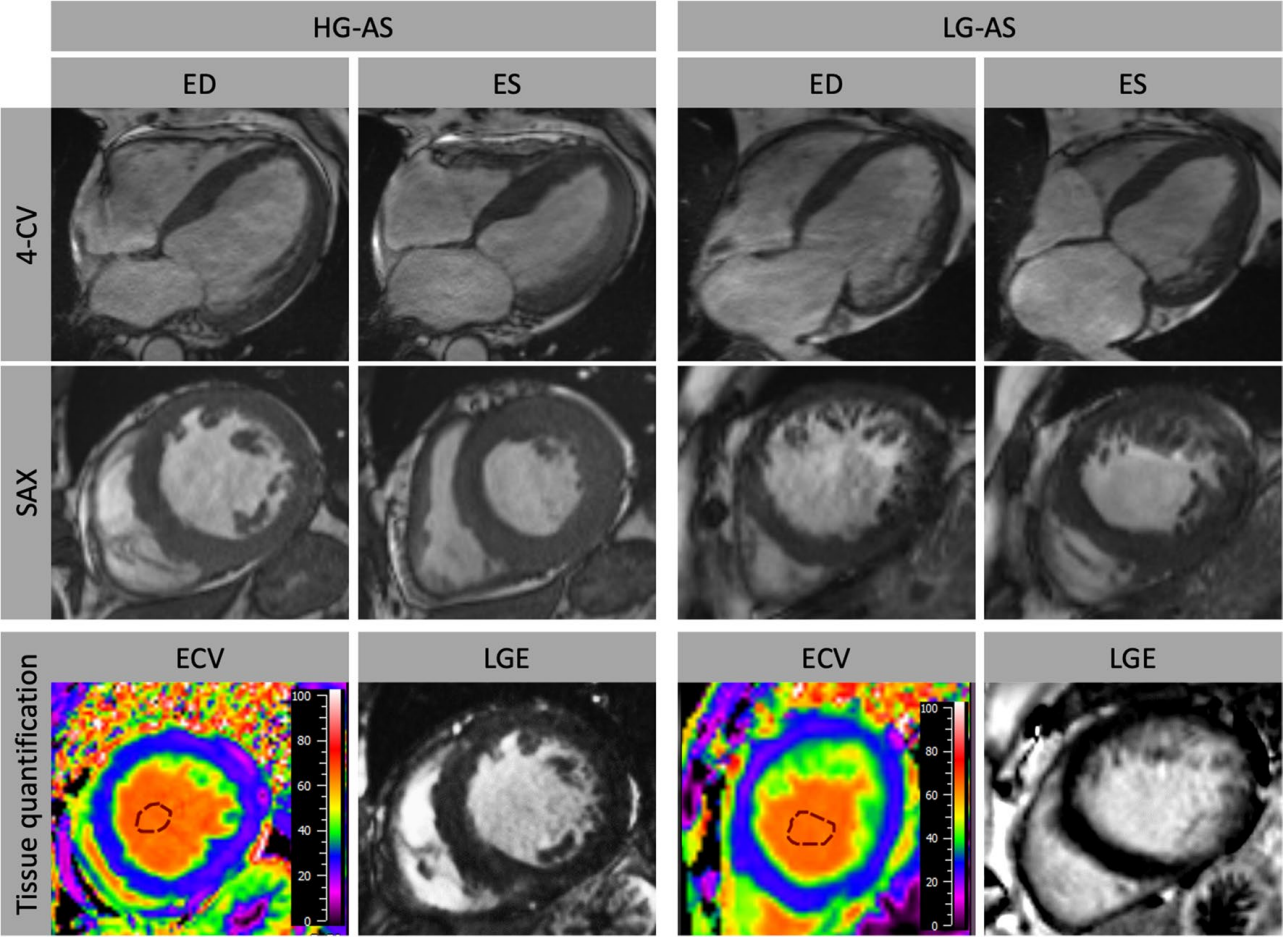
Cardiovascular magnetic resonance imaging in patients with high-gradient (HG) and low-gradient (LG) aortic stenosis (AS). Myocardial function was analyzed in long-axis orientation including 4-chamber view (CV) as well as short-axis (SAX) orientation. Furthermore, tissue characterization comprising calculation of extracellular volume (ECV%) and late gadolinium enhancement (LGE) was performed. ED: end-diastole; ES: end-systole
